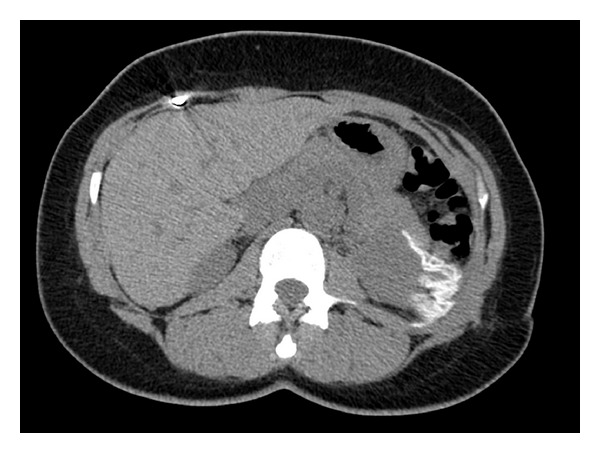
Postoperative CT abdomen demonstrating PTFE bolster on left kidney.
